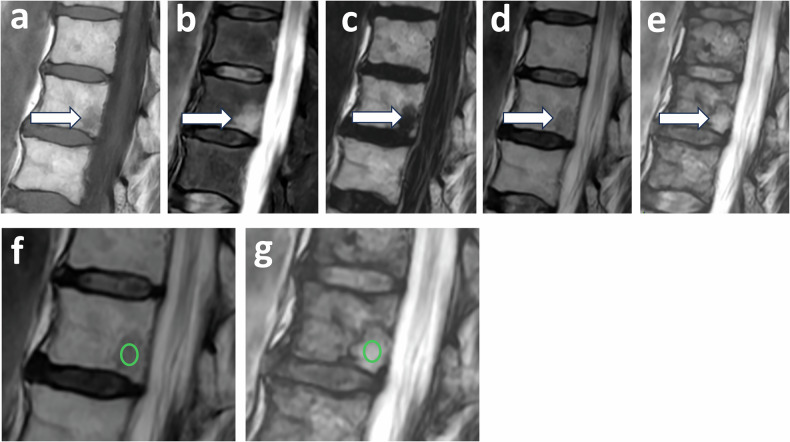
Differential diagnosis between metastasis and benign nodular marrow hyperplasia. Metastasis in a 72-year-old male with newly diagnosed prostate cancer. Sagittal spin echo T1-weighted (a), and fast spin echo T2 Dixon water-only (b), fat-only (c), in-phase (d, f) and out-phase (e, g) images. A focal lesion (arrows) shows low signal on T1 (a) and fat Dixon (c) images, high signal on water-only Dixon (b). The lesion signal shows no significant signal drop (1.3%) on the out-phase image (arrow in e; ROI with measured signal intensity in g = 588) compared to the in-phase image (arrow in d; ROI with measured signal intensity in f = 596), indicating the disappearance of marrow fat due to its replacement by tumoral tissue. These characteristics allow the confident diagnosis of a bone marrow metastasis. The diagnosis was confirmed by a lesion increase in size and the appearance of new metastases at imaging follow-up
